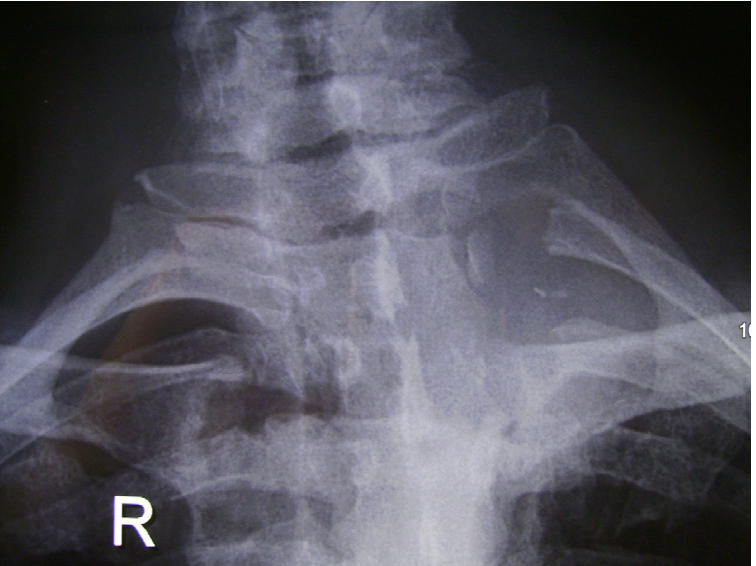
Post-operative chest X-ray demonstrating removal of the T2 and T3 transverse processes, and proximal parts of the second and third ribs.
